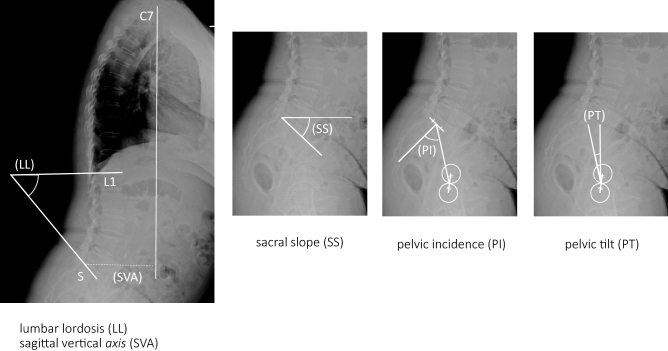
Radiographic measurements of spinal sagittal parameters. Image shows the lumbar lordosis (LL) which is the angle between the upper endplate of L1 and S1, the pelvic tilt (PT) which is the angle between the line drawn through the center of the femoral head and the midpoint of the sacral plate and the vertical reference, the pelvic incidence (PI) which is the angle between the line through the center of the femoral head and the midpoint of the sacral plate and the line perpendicular to the sacral plate, the sacral slope (SS) which is the angle between the horizontal line and upper endplate of S1, and the sagittal vertical axis (SVA) which is the distance between the C7 plumb line and the posterosuperior aspect of S1.
